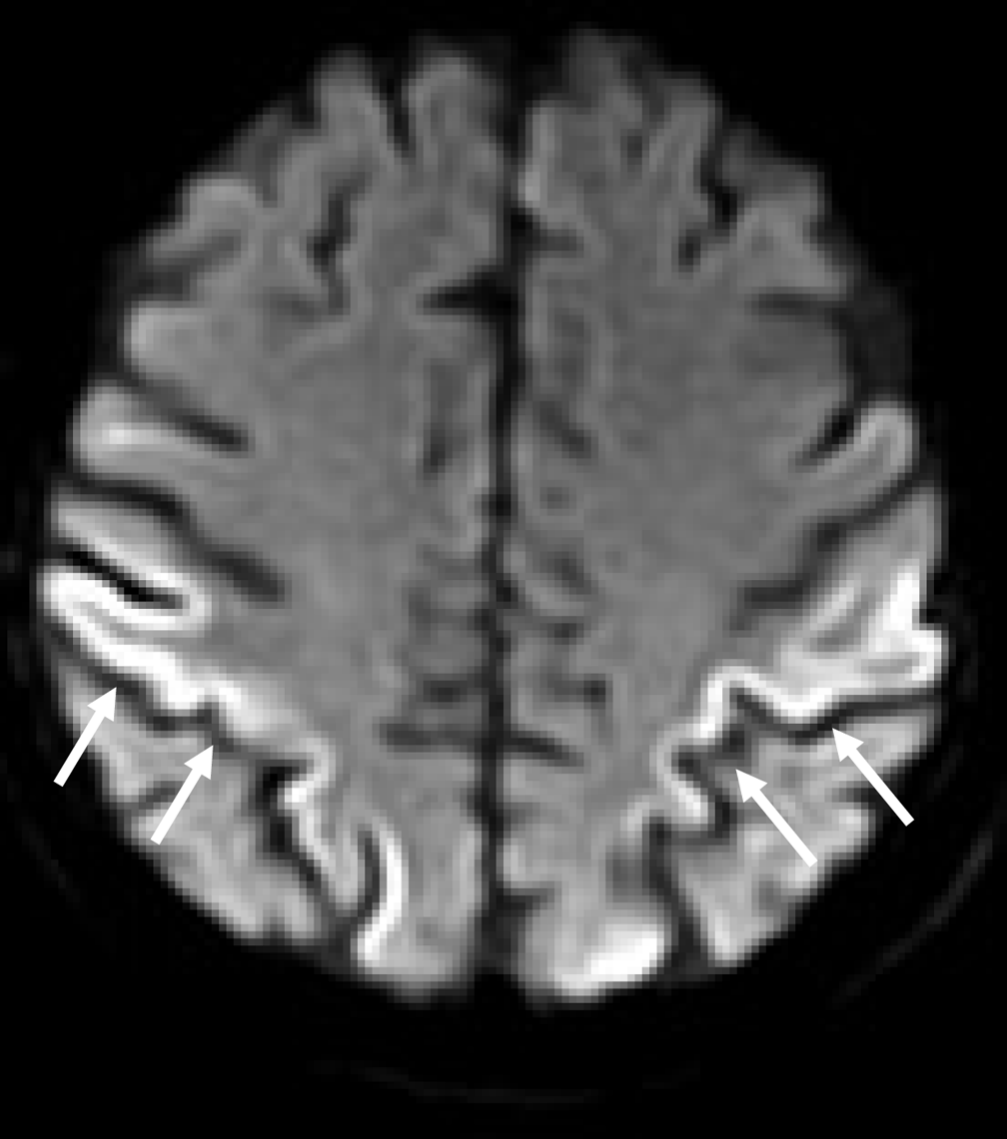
MRI taken upon admission of a 76-year-old woman with pronounced dressing apraxia. Diffusion-weighted imaging showing hyperintense, ribbon-shaped signal irregularities predominately in the parietal cortex bilaterally (white arrows).
